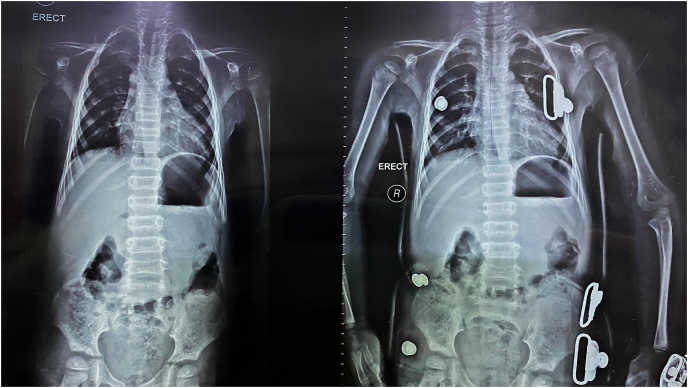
A spine X-ray in the anterior-posterior position showing the improvement in the spine deformities after using a back brace.
